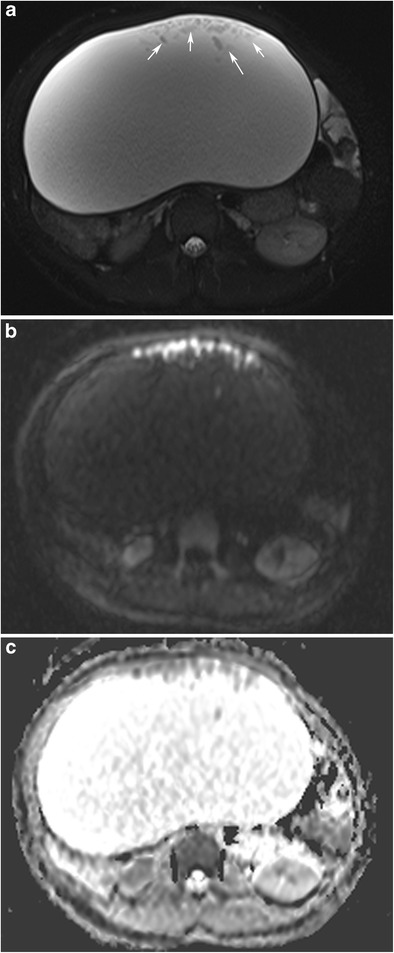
Axial fat-saturated T2-weighted (a), diffusion-weighted (*b 800*) image (b) and ADC map (c) images of a 14-year-old female with a mature cystic teratoma. Multiple floating structures (arrows) are seen in the anterior part of the cystic mass. Those structures show diffusion restriction regarding the keratin content, which was also proven pathologically
